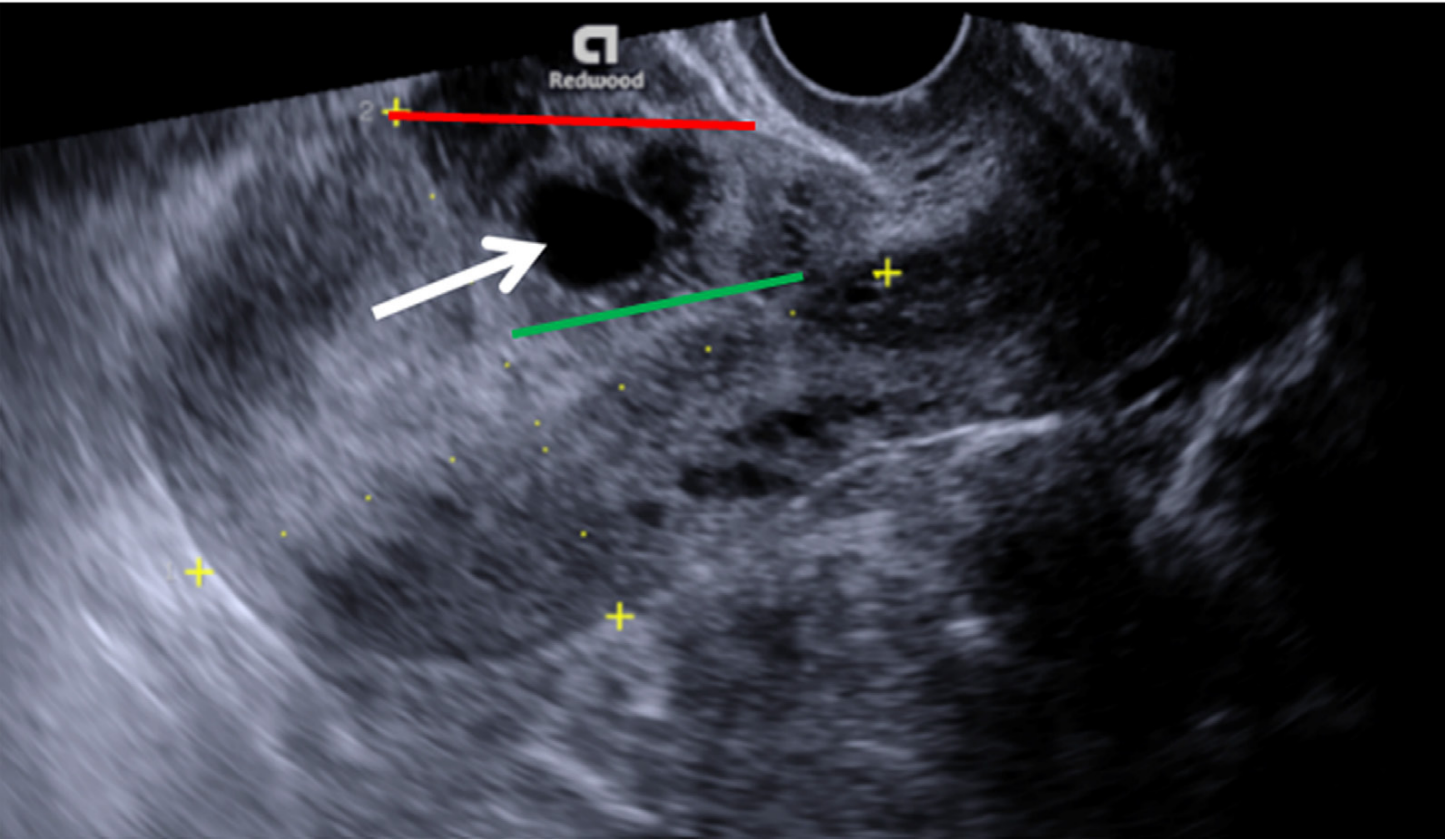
Transvaginal grayscale ultrasound image of the uterus in sagittal plane demonstrates gestational sac (arrow) implanted in the niche of previous caesarean scar site, crossing serosal line (red line), while uterine cavity line (green line) remains intact
